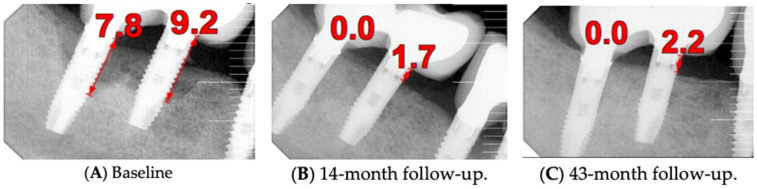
Periapical images showing the evolution of the case (red arrows show the distance from the implant platform to the bone—(A) Baseline; (B) After 14 months; (C) After 43 months).
